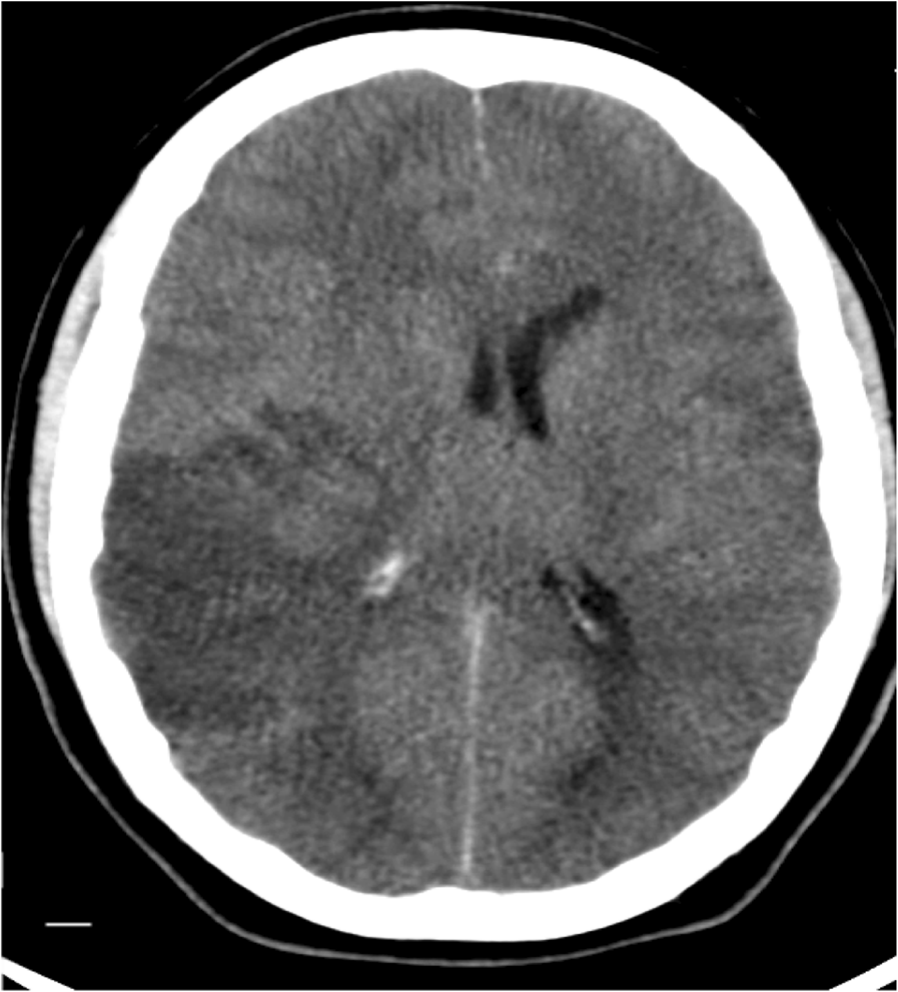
Computed tomography (axial) demonstrating a large middle cerebral artery infarct and concomitant perilesional edema
